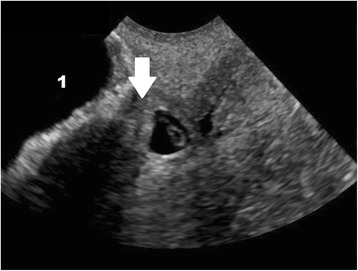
Cesarean scar ectopic pregnancy by transvaginal ultrasound. The arrow indicates the thin myometrium (3 mm) between the bladder (indicated with the number 1) and the gestational sac
